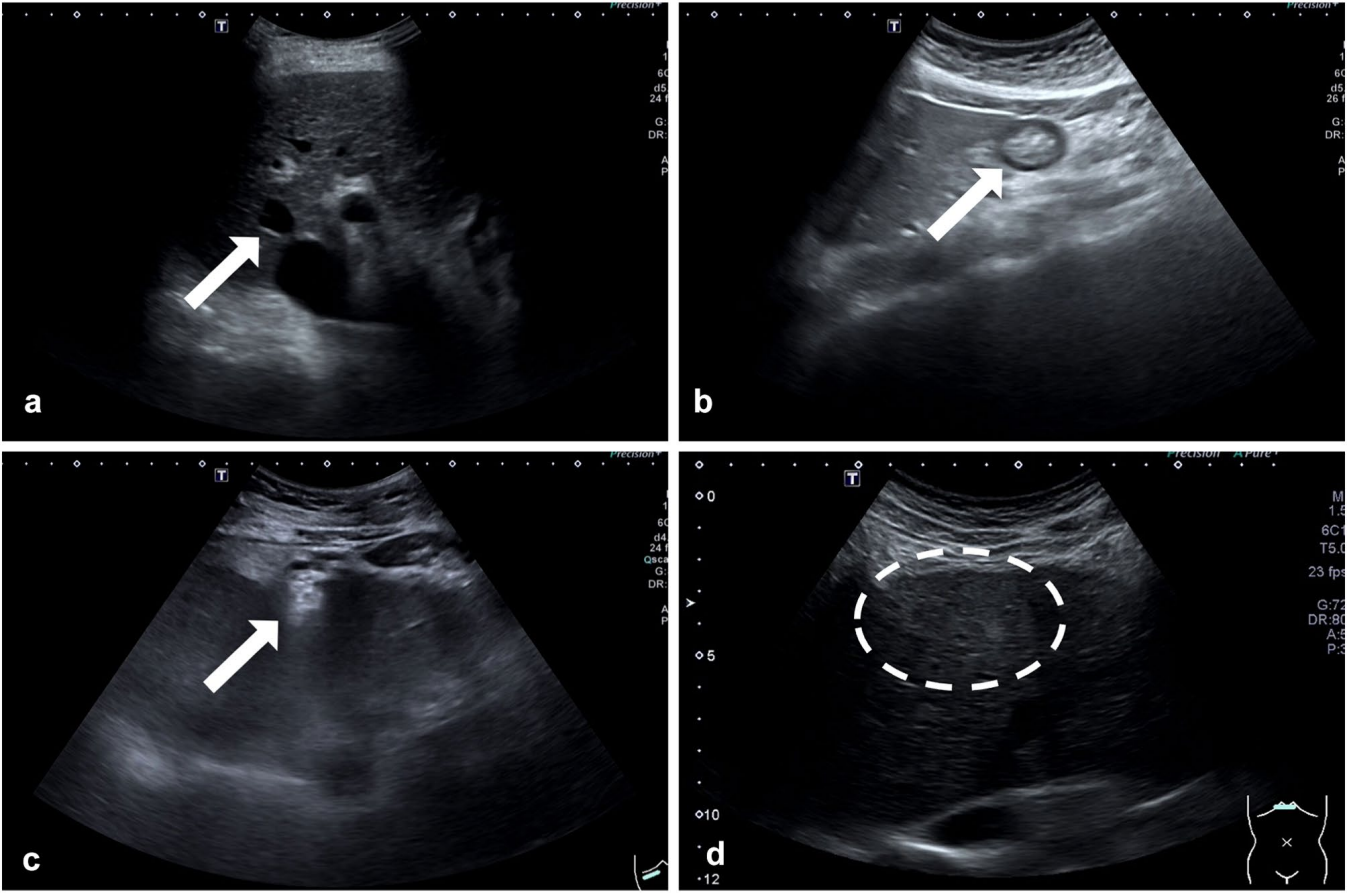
Difficult frames in Group FP used to train the AI system in the Step 2 Training. These are frames in which Model B pre-trained on ultrasound snapshot images in the Step 1 Training falsely detected other structures (arrows) as FLLs (a: blood vessel, b: stomach, c: fibrous tissue, d: parenchymal heterogeneity (dashed circle)).
